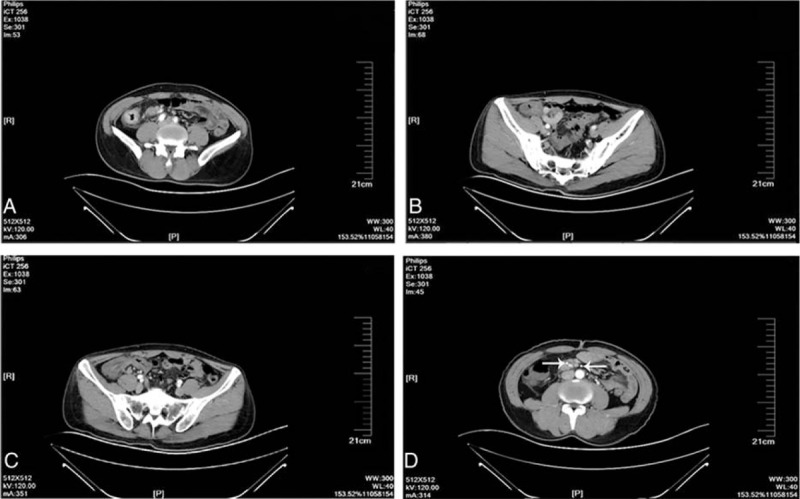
Enhanced abdominal computed tomography images. (A) Ascending bowel wall thickening; (B) cecum and ileum wall thickening; (C) normal mucosa between the colon and appendix; and (D) ileocolic artery and middle colic artery, and right colic artery was absent.
